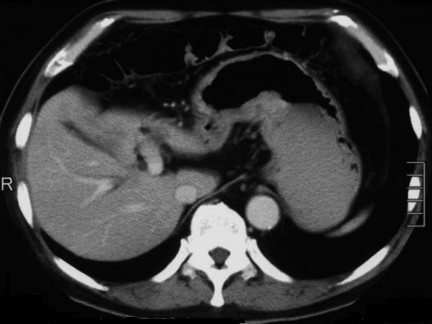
Abdominal computed tomography showing a thickened posterior wall of the gastric body. No evidence of lymph node swelling or a metastatic liver tumor is observed.
